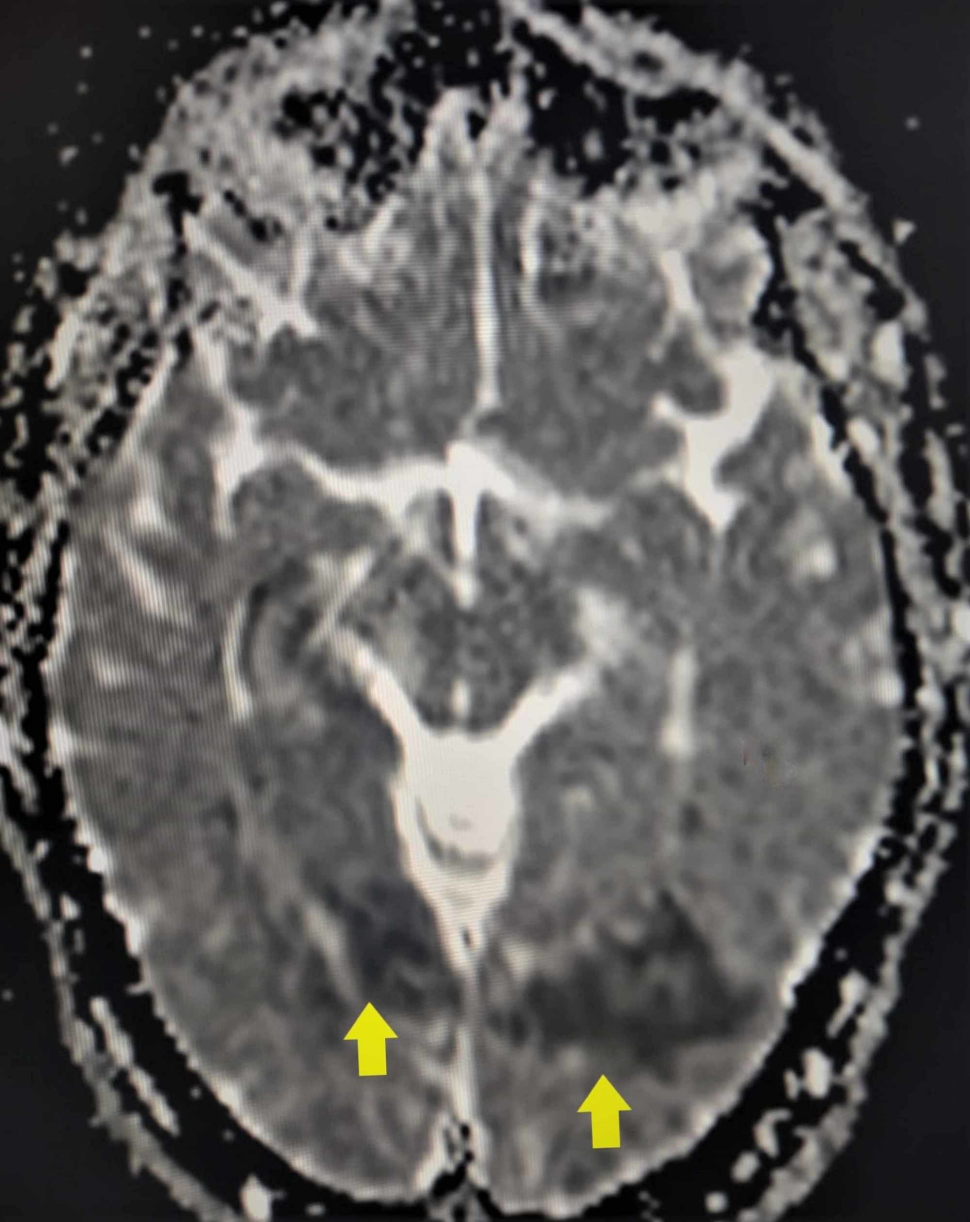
DW/ADC MRI sequence The image shows areas of patchy diffusion restriction (yellow arrows) MRI: magnetic resonance imaging; DW: diffusion-weighted; ADC: apparent diffusion coefficient
